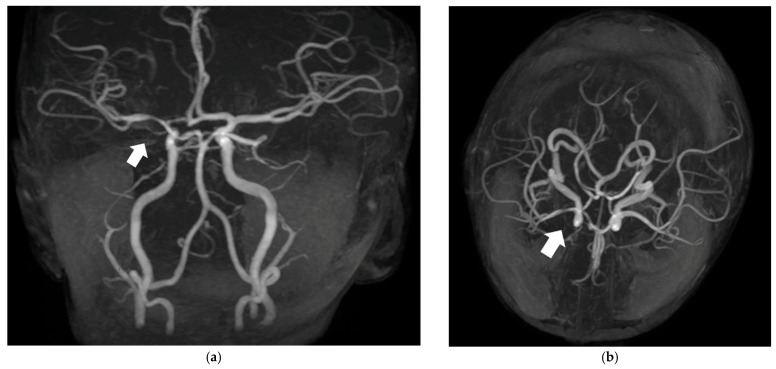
Brain magnetic resonance imaging (MRI). (a) Coronal angiographic sequence showing focal abnormalities, suggestive of vasculitis, of the proximal M1 segment of the right middle cerebral artery (arrow). (b) Axial angiographic acquisition showing focal involvement, suggestive of vasculitis, of M1 segment of the right middle cerebral artery (arrow).
